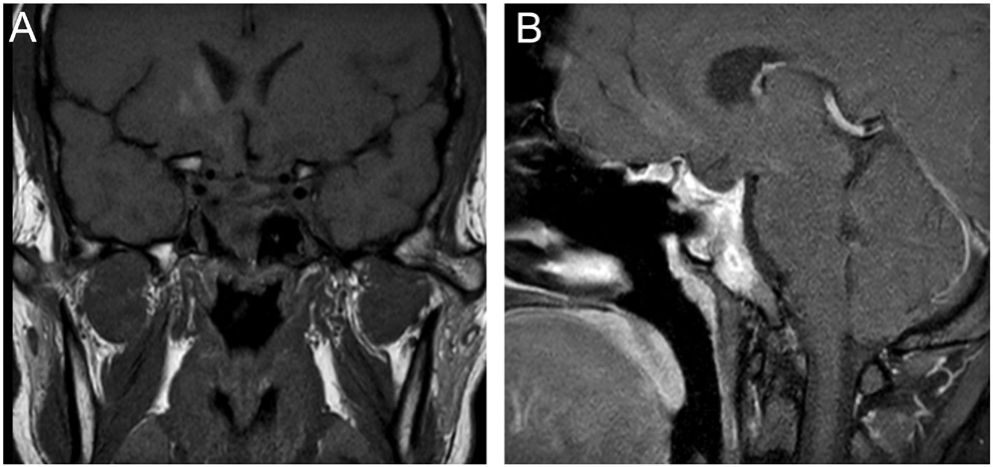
(A) T1-weighted coronal image pre-contrast – previously seen rim-enhancing lesion is not seen in this study. (B) T1-weighted sagittal image shows residual peripherally enhancing lesion at the body of clivus.
